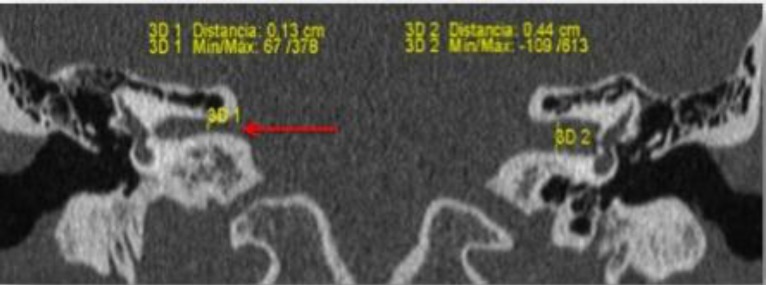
Coronal CT image through the inner ear and inner auditory canal shows a narrow width of right inner auditory canal (red arrow) in comparison to the left
